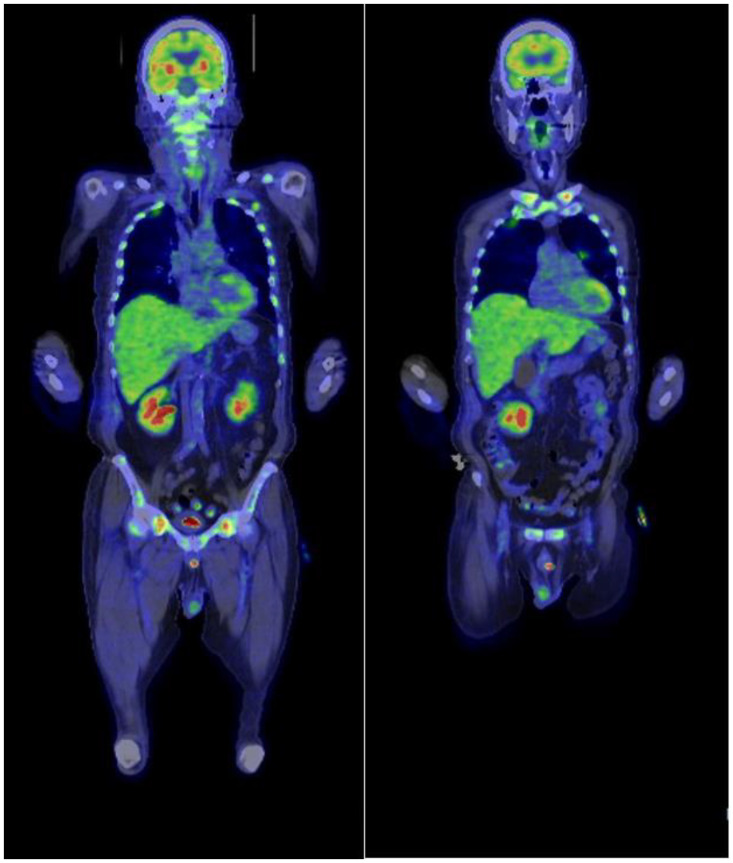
A lesion exhibiting high 18-FDG uptake (SUVmax, >4) was detected in the bones (clavicle, humerus, spine, rib, pelvis, and femur) and spleen. 18-FDG, 2-deoxy-2-(fluorine-18)-fluoro-D-glucose; PET, positron emission tomography; SUVmax, maximum standard uptake value.
